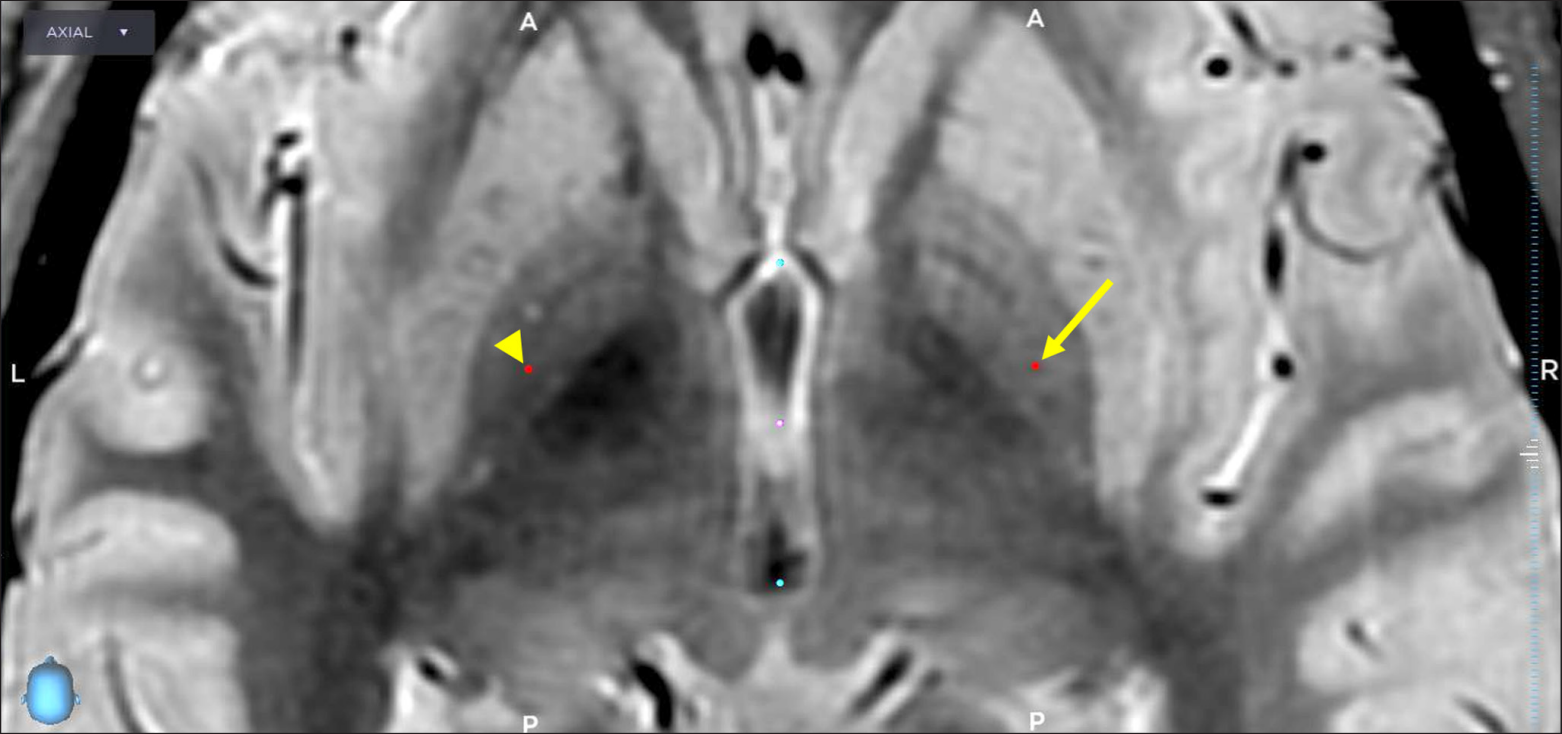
Proton density weighted images obtained on a 3-Tesla MRI demonstrates the anatomical target on the left (arrowhead) and right (arrow) corresponding to Talairach coordinates of (–21, 4.5, 0) and (21.25, 4.75, 0) respectively.
